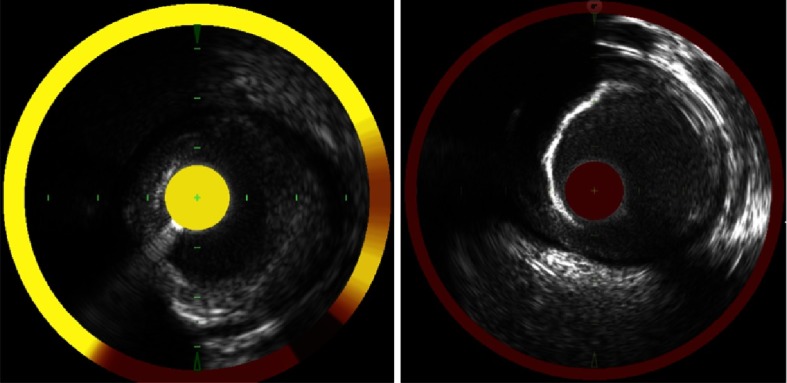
Combined NIRS-IVUS images. The current clinically available NIRS device provides combined automatically co-registered NIRS and IVUS images that are obtained during a single pullback within a coronary artery. Shown are two examples of combined NIRS-IVUS images. In each image the NIRS chemogram is located around the periphery of the greyscale IVUS image, whereas the block chemogram is shown in the central circle. Left: A plaque having a moderate plaque burden by IVUS is composed of lipid as demonstrated by the NIRS chemogram and block chemogram, both of which reveal yellow signal. Right: A calcified plaque by IVUS is shown to be devoid of lipid by NIRS, as both the chemogram and block chemogram are red. IVUS = Intravascular ultrasound; NIRS = near-infrared spectroscopy.
